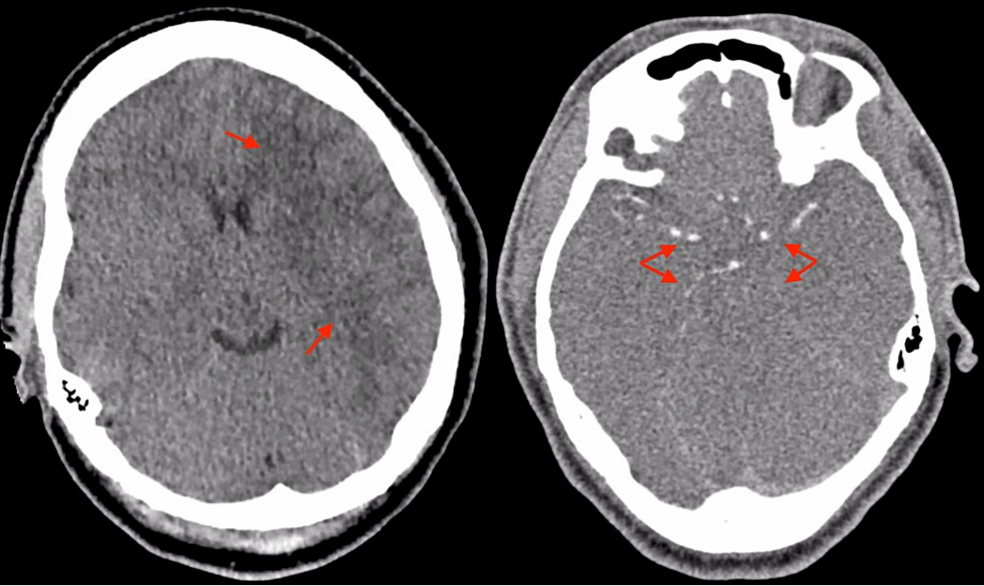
The CT and CT angiogram of the head upon admission Left: The CT scan of the head revealed infarction in the middle cerebral artery territory, with the left hemisphere (Arrows) affected more than the right.
